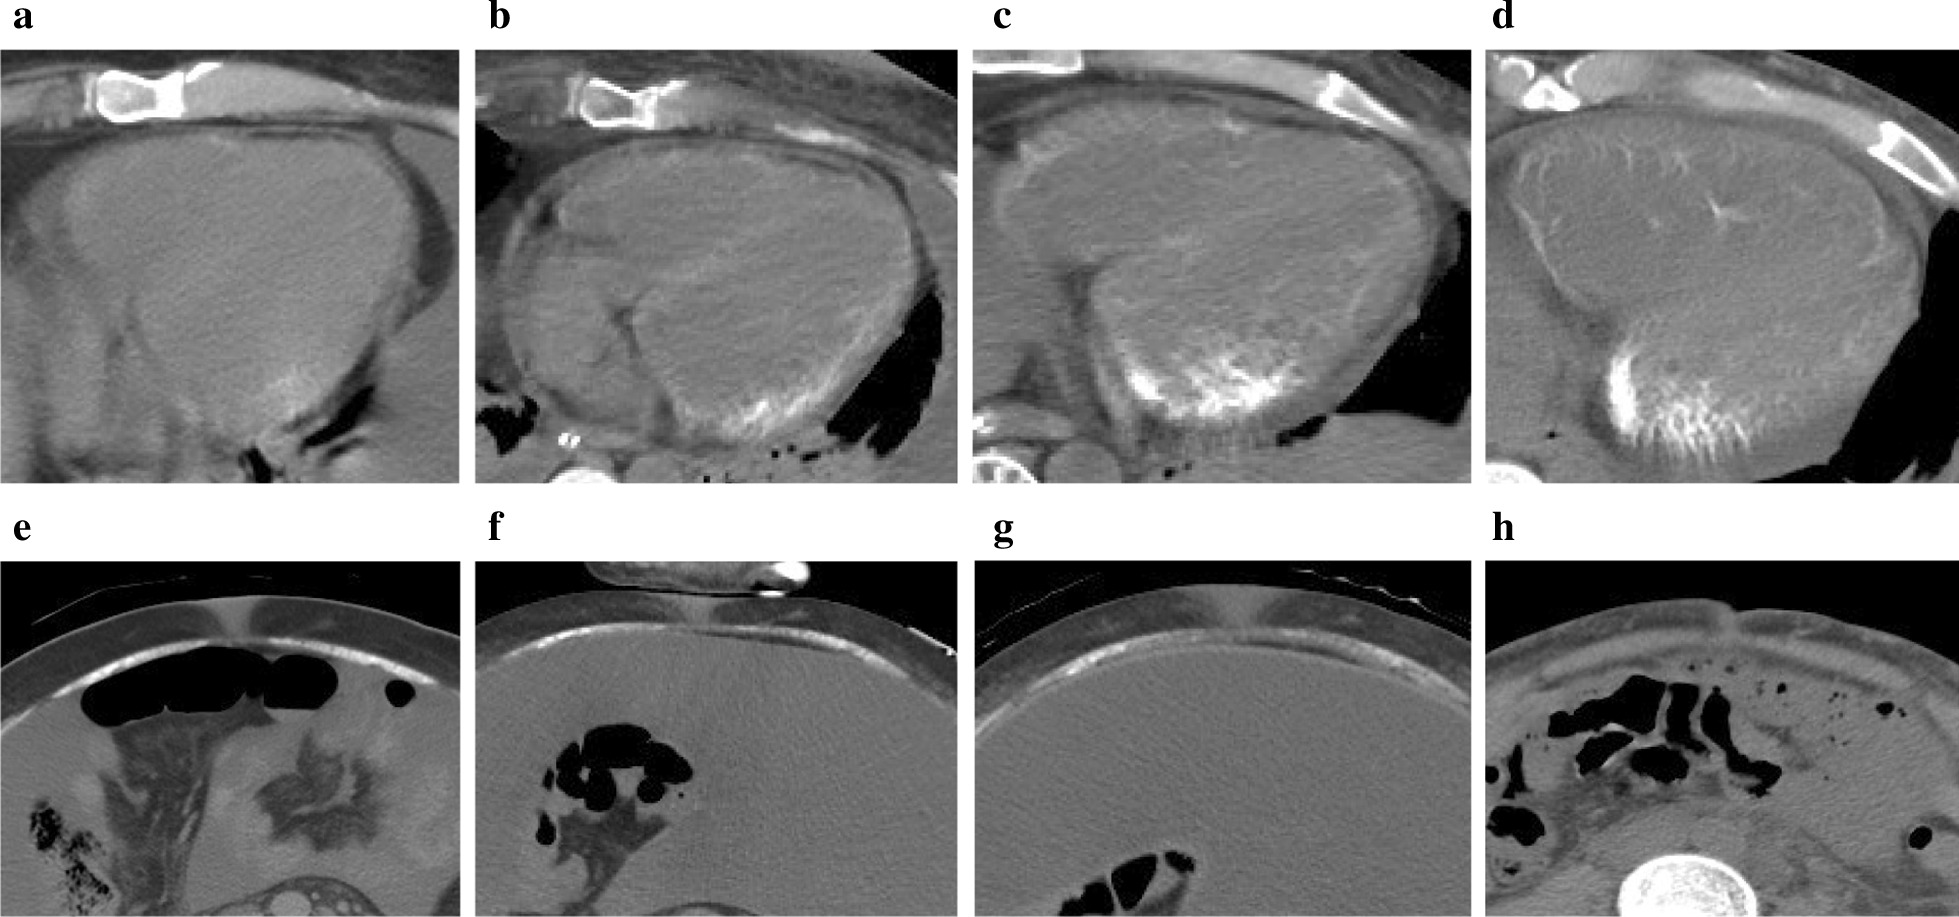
Chronological changes in myocardial and skeletal muscle (rectus abdominis) calcification on computed tomography images. Computed tomography was performed on (a, e) day 47, (b, f) day 123, (c, g) day 177 and (d, h) day 345. The degree of calcification showed progressive worsening during the first few months; subsequently, myocardial calcification remained unchanged, while that in the skeletal muscle gradually diminished
